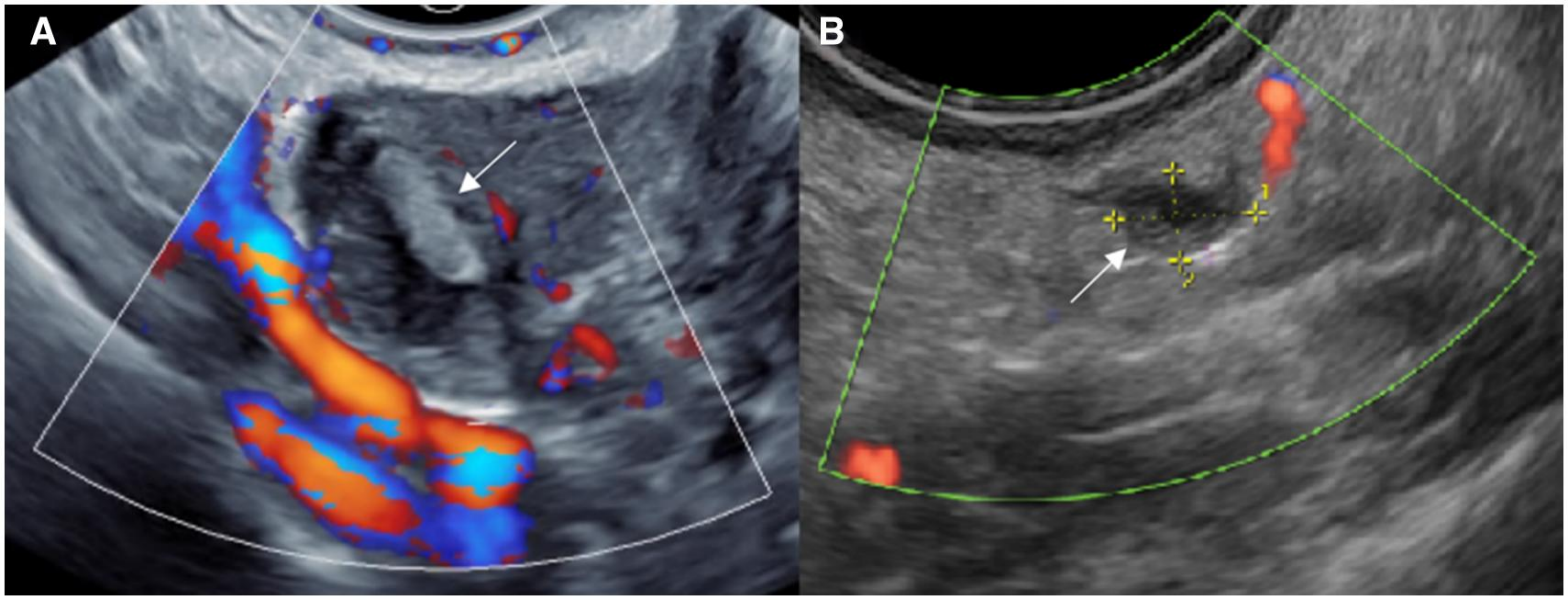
A 44-year-old female underwent a pelvic ultrasound for investigation of heavy menstrual bleeding. Transvaginal ultrasound revealed multiple bilateral endometriomas with fluid-fluid levels consistent with bleeding occurring at different time points and right uterosacral DE. (A) Right ovarian endometrioma containing avascular echogenic internal material (arrow), in keeping with blood products. (B) Right uterosacral ligament endometriotic deposit (arrow), consistent with DE.
